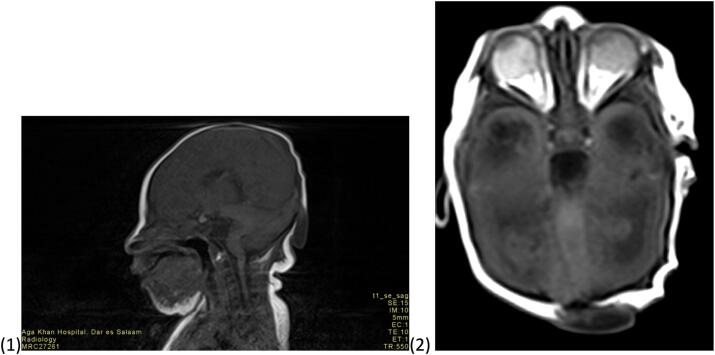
Multiplanar multisequence MRI was performed on the brain in standard orthogonal planes. MRI brain T1 sagittal and axial images showing the occipital encephalomeningocele with small part of right cerebellar lobe, meninges and CSF herniating into it.
